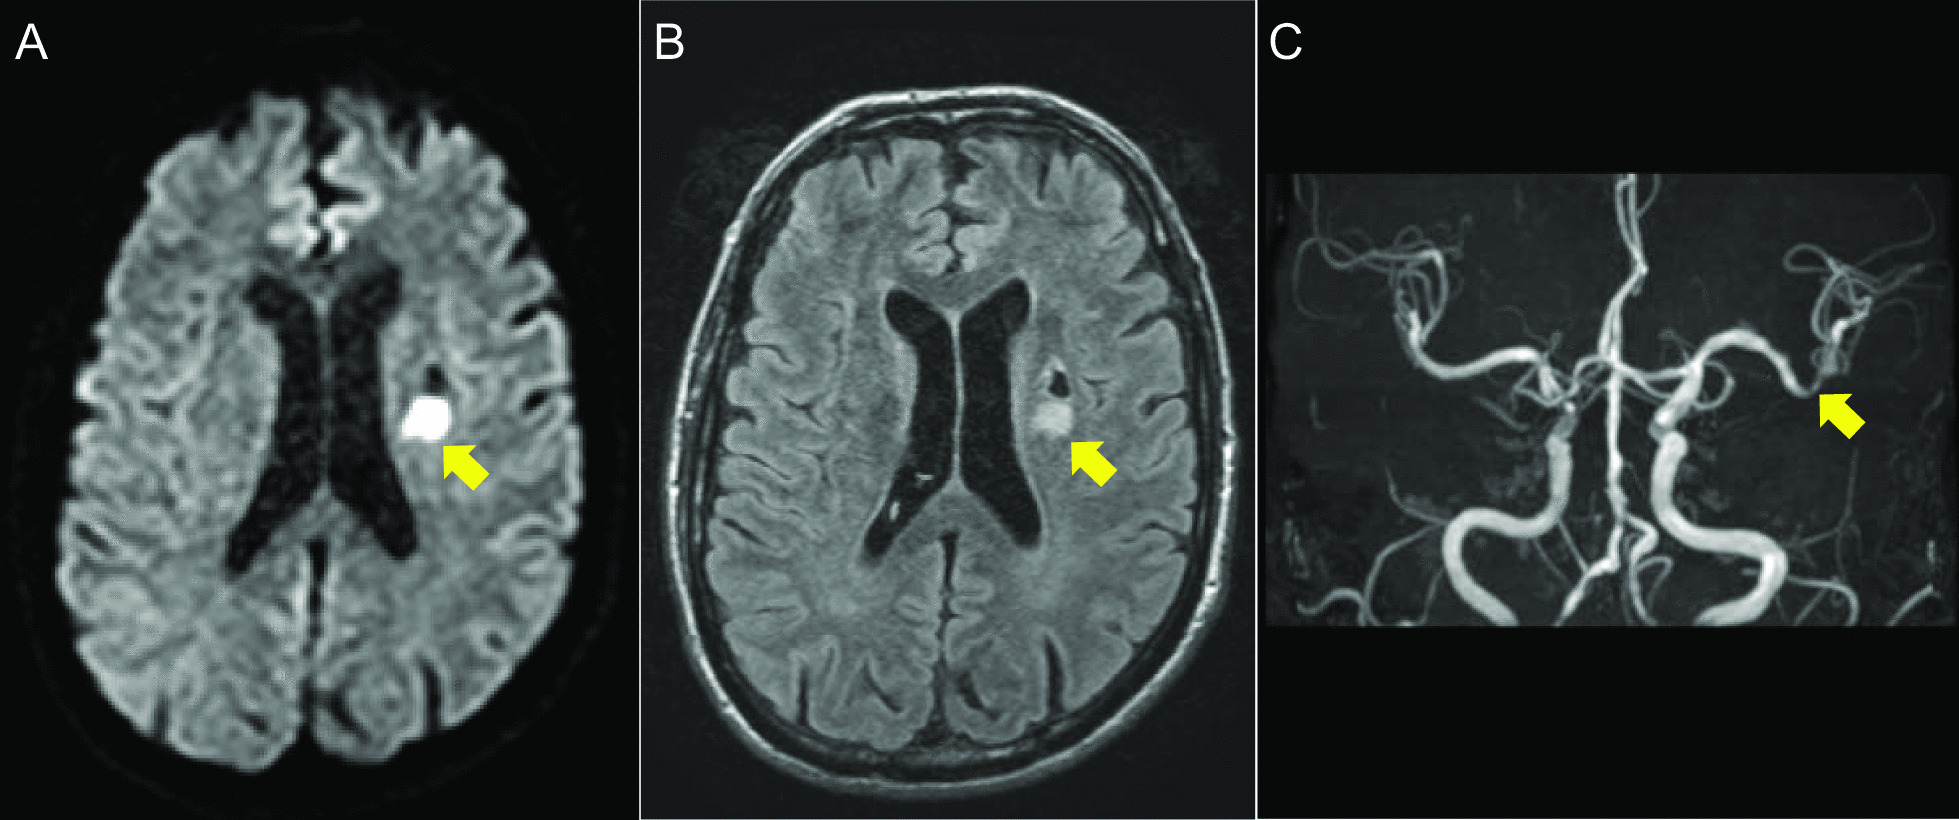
Magnetic resonance images of the brain after 8 days of removal of Impella device. A Axial diffusion-weighted MRI showing hyperintensity in the corona radiata (arrow). B Axial T2-weighted FLAIR MRI showing similar findings (arrow). C Frontal view from magnetic resonance angiography showing stenosis of the left middle cerebral artery (arrow)
